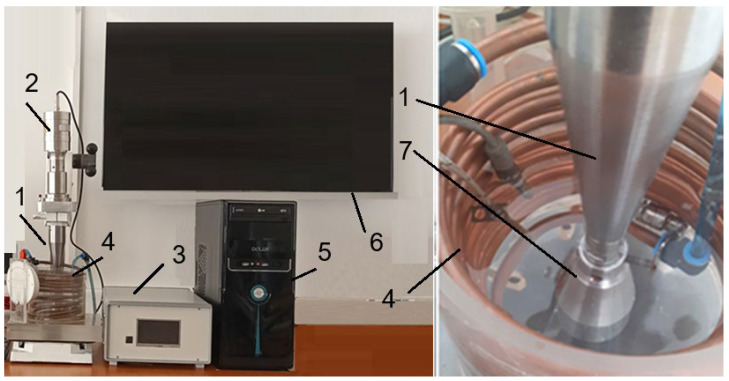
Cavitation testing apparatus: 1—sonotrode; 2—piezoceramic transducer 20 kHz; 3—electronic ultrasound generator; 4—the vessel with liquid and the cooling coil; 5—computer for controlling functional parameters; 6—monitor for tracking operating parameters; 7—fixing device for sample.
